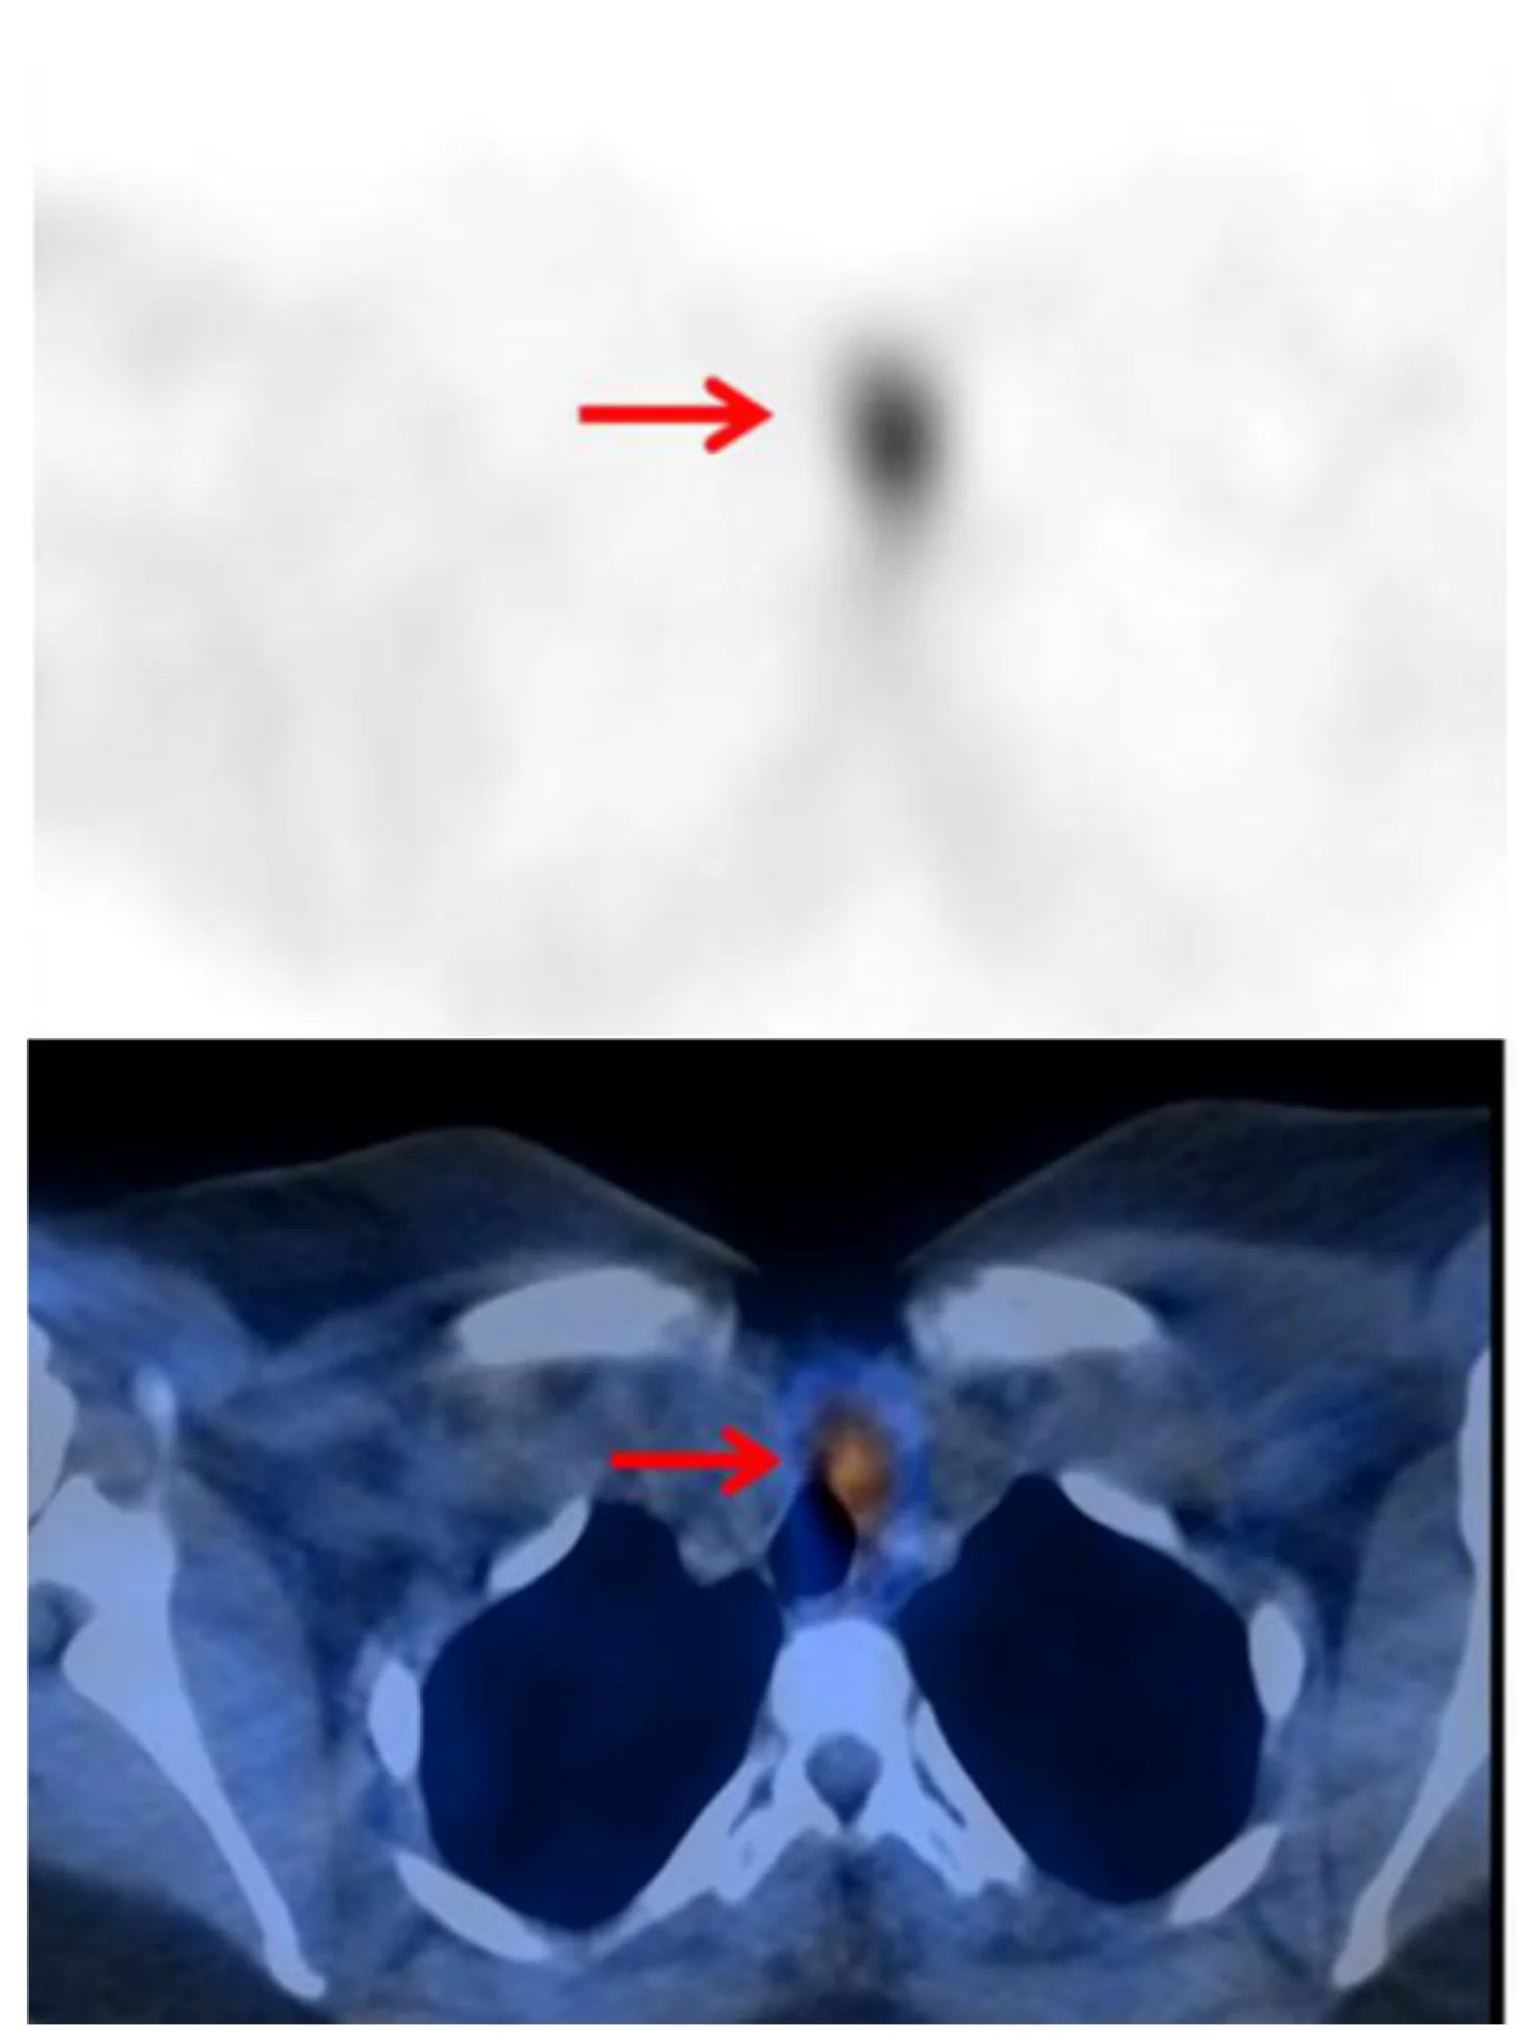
SPECT (upper panel) and fused SPECT/CT (lower panel) of [^99m^Tc]Tc-MIBI in a patient with left PA (red arrow).
